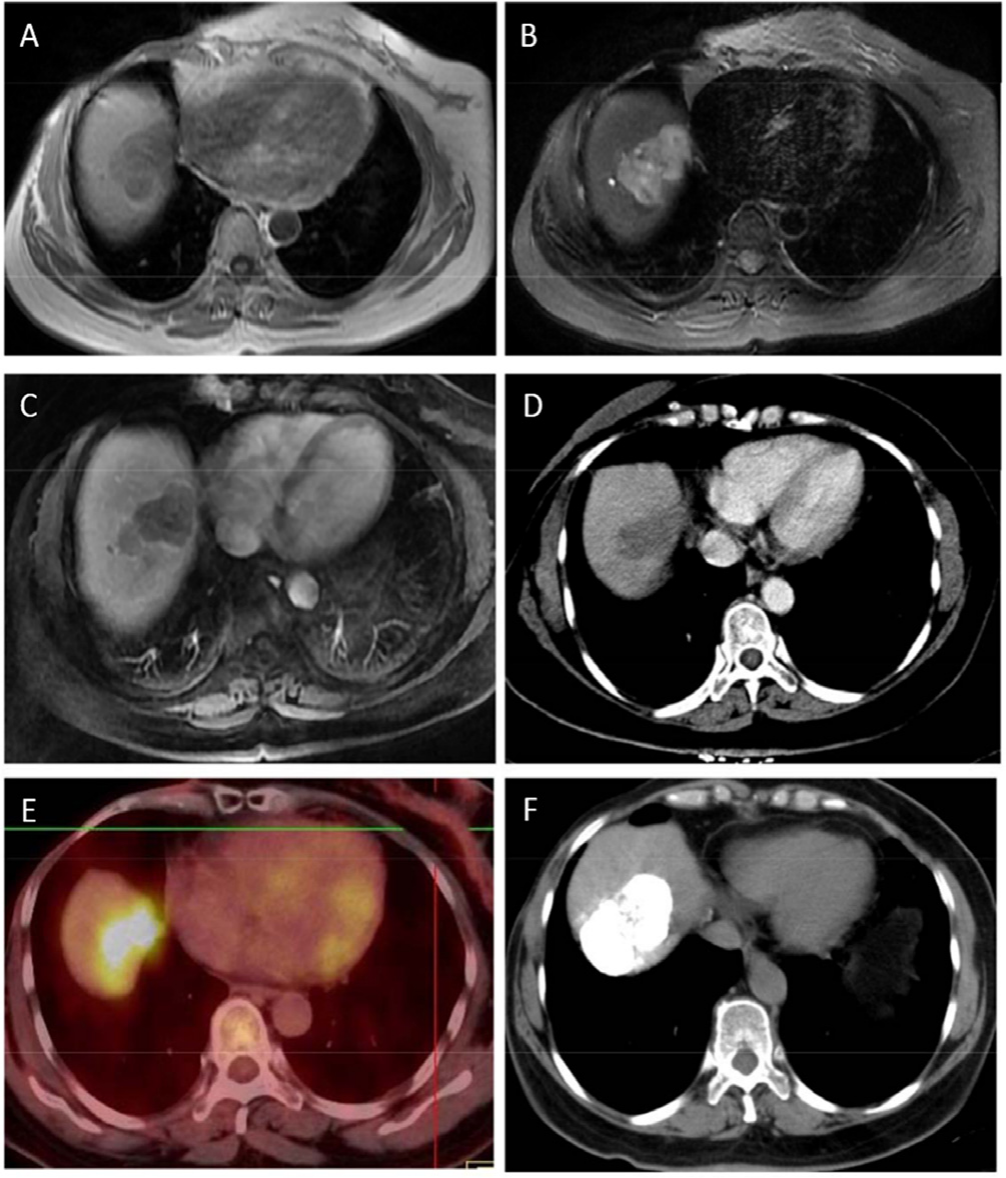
A: T1 weighted MR image shows irregular and clear border of the lesion with low signal. B: T2 weighted MR image shows high signal of the lesion with inhomogeneous signal inside. C: Late arterial phase of dynamic contrast enhanced scan shows central lesion without obvious enhancement, but part of the border has ribbon-like enhancement. D: Portal venous phase of dynamic contrast enhanced scan shows central lesion still has no enhancement and the previous enhancement part hasn’t washed out. E: PET-CT shows abnormal increase of radioactivity uptake with SUV max 6.9. F:CT scan shows good lipiodol deposition after transarterial chemoembolization.
